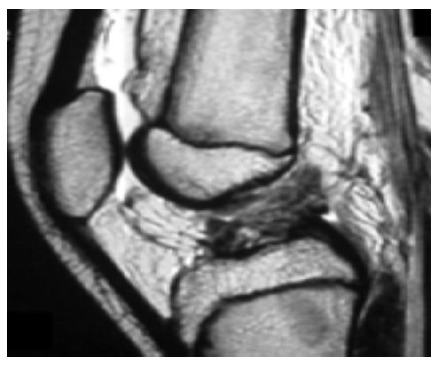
Sagittal MRI showing a complete ACL tear in a pediatric patient.
